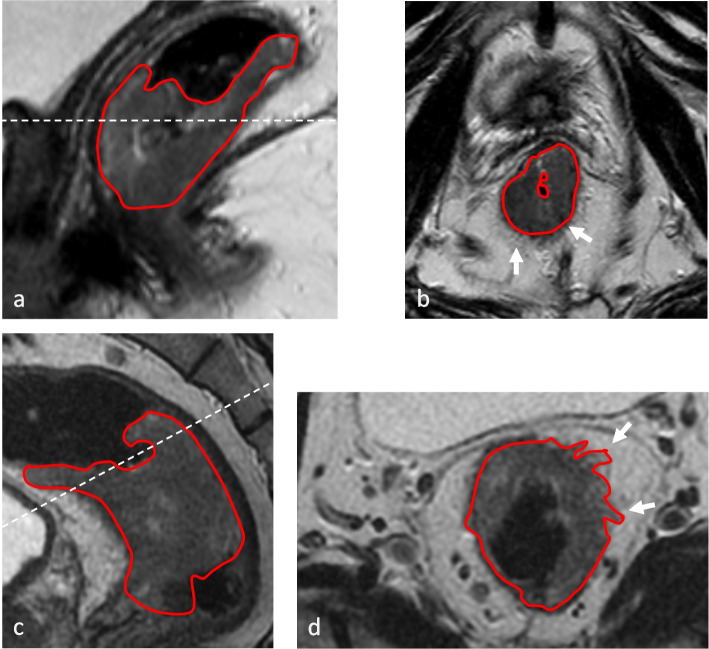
a–d Sagittal and axial (oblique) T2-weighted MR images from two patients from different centres. Both these investigations were performed in the baseline setting; therefore, differences in image quality are irrespective of the effects of neoadjuvant treatment. Tumour borders are delineated with continuous red lines. White dashed line in a and c = plane of the axial MR image shown in b and d. a, b Both the sagittal and the axial sequences had a slice thickness of 4 mm. In both images, the rectal wall is not clearly defined. b Axial projection of the tumour. Structures in the mesorectal fat are not clearly visible. The image of the invasive front is blurred (arrows). No sequence perpendicular to the tumour was obtained for this patient. c, d Both the sagittal and the axial oblique sequences had a slice thickness of 3 mm. In both cases, the rectal wall is clearly defined, and invasion of the mesorectal fat is distinct. d The invasive front is indicated by white arrows
